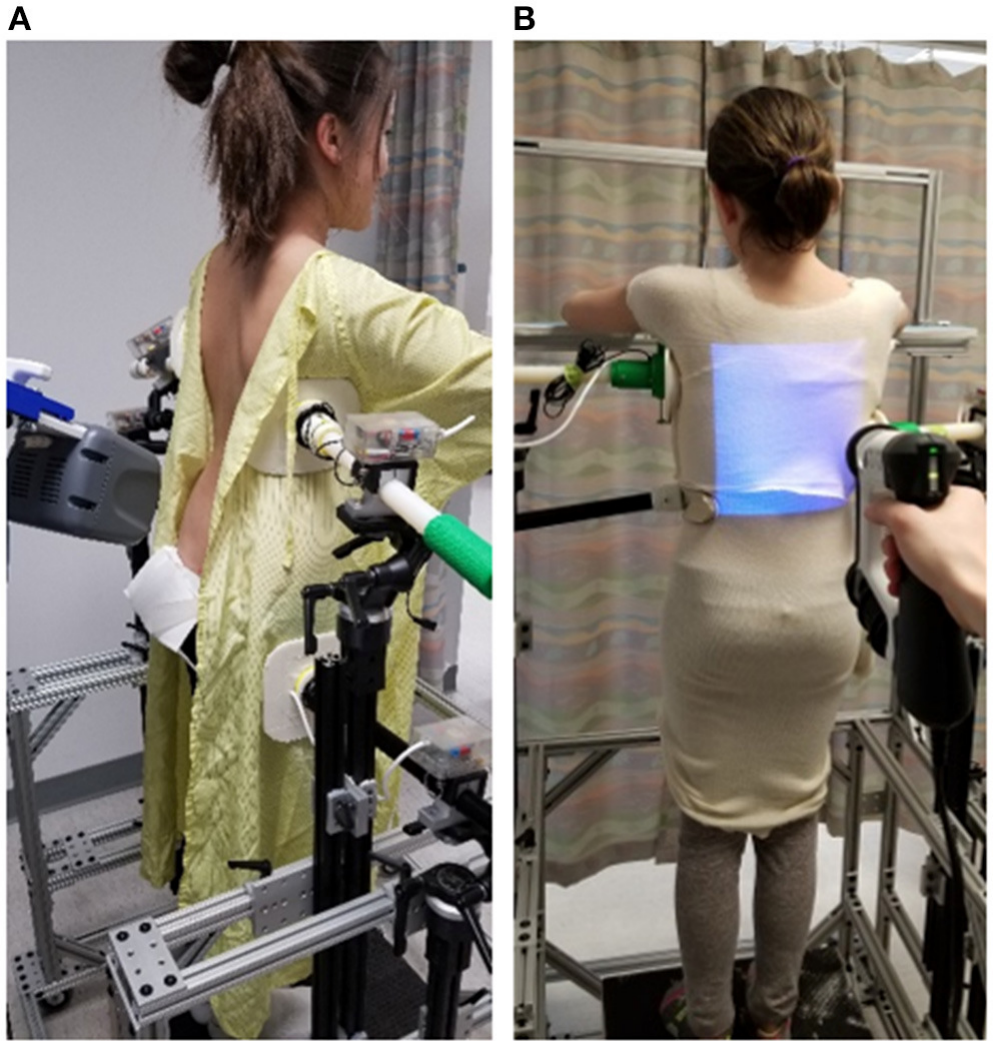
A participant standing inside a custom frame (A) for ultrasound assisted brace casting, (B) for body scan using a 3D Spectra Scanner.
